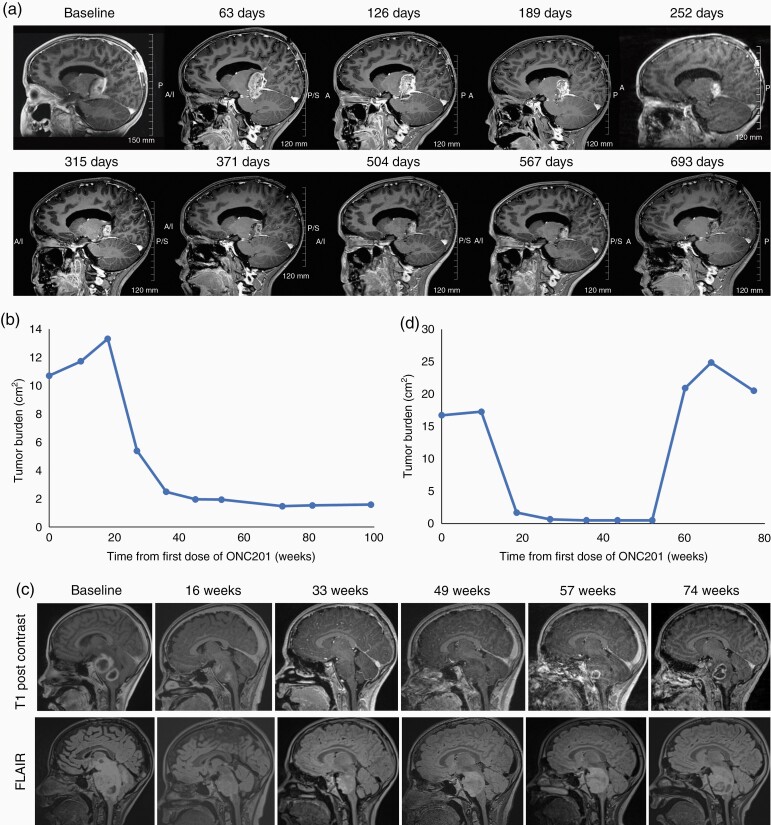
Radiographic evaluation of ONC201 in pediatric patients with H3 K27M-mutant thalamic glioma (a, b) or DIPG (c, d) who had previously received radiation. (a) Sagittal T1 post-contrast MRI images and (b) tumor size in enrolled patient prior to and following ONC201 treatment. Baseline MRI is 2 days after completion of radiation and ONC201 treatment began 7 days after completion of radiation. (c) Sagittal T1 post-contrast and T2/FLAIR MRI images of the primary tumor at baseline and at 19, 36, 52, and 60 weeks after beginning ONC201 treatment of a 9-year-old male with DIPG who began ONC201 4.5 weeks after completing radiation therapy and (d) radiographic change in tumor burden from baseline.
